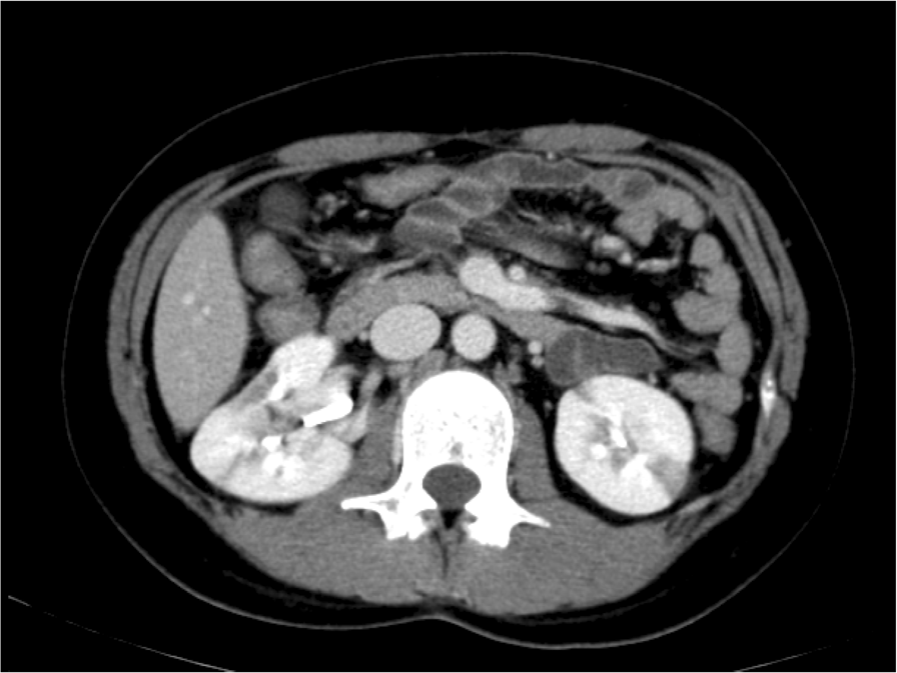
Findings on abdominal contrast computed tomography. Regions with poor contrast enhancement are noted in both kidneys
